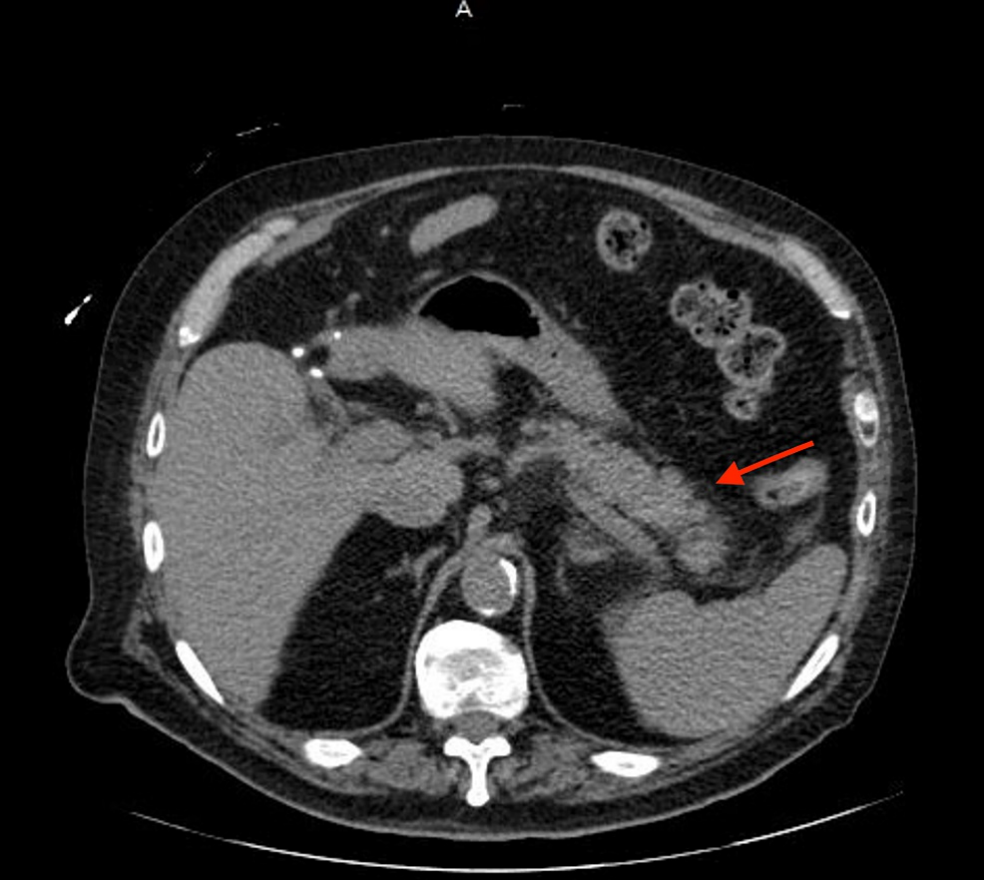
Computed tomography scan of the abdomen/pelvis demonstrating findings associated with acute pancreatitis (red arrow) including diffuse enlargement of the pancreas in addition to an irregular contour of the pancreatic margins, and peripancreatic fat stranding.
